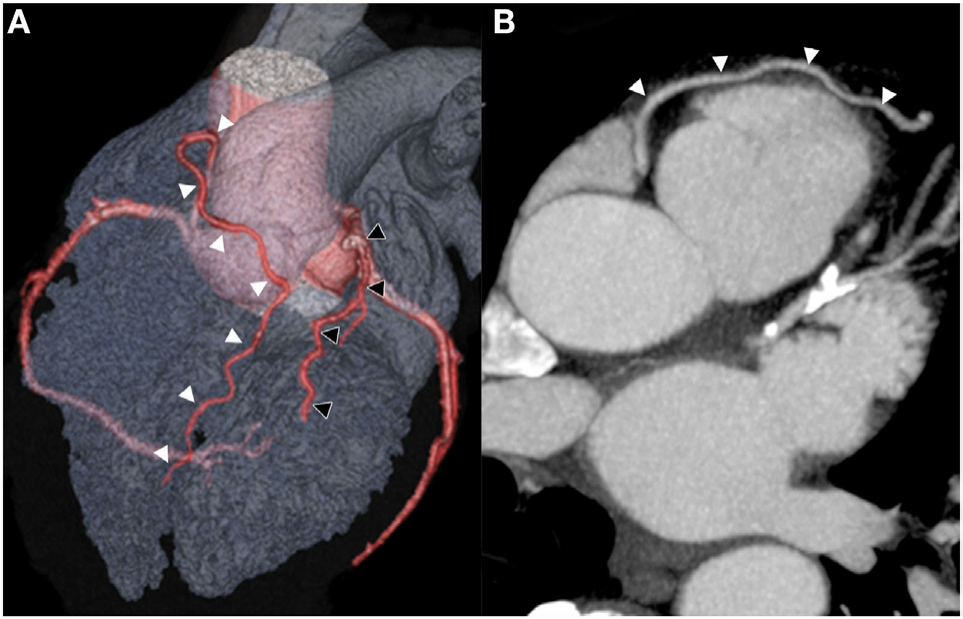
An anterior projection of a three-dimensional volume-rendered computed tomography image confirms the short LAD (black arrowheads) originating from the left main coronary artery terminating its course in the interventricular sulcus. Additionally, a long LAD (white arrowheads) is visualized originating above the right coronary artery sinus initially pointed cranially, then traveling anteriorly to the interventricular sulcus and finally descending toward the apex (A). Axial computed tomography image demonstrates a long LAD epicardial course (arrowheads) as it passes anteriorly to the right ventricular outflow track, consistent with a Spindola-Franco class IV dual LAD (B).
